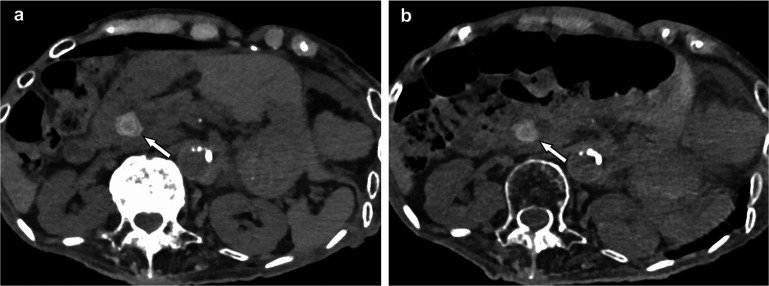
Non-contrast abdominal CT images of a patient with choledocholithiasis scanned with PCCT (A) and conventional DSCT in single-energy mode (B), performed on different days. The PCCT image, with a CTDIvol of 7.5 mGy, demonstrates reduced noise, enhanced image quality, and improved visualization of small structures, such as the calcified stone in the common bile duct (arrows), compared to the conventional CT image, which had a CTDIvol of 11.9 mGy. Reproduced from Onishi *et al* (2024). CC BY 4.0.
